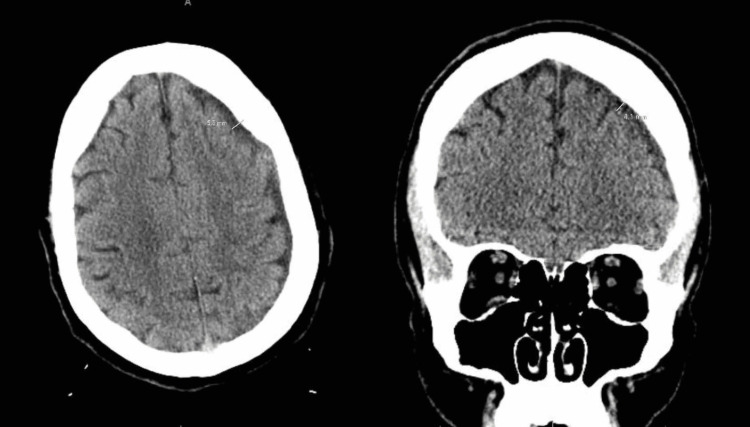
CT of the head without contrast showing changes from January to the follow-up in March. The interval decreased from 5.6 mm to 4.1 mm.
